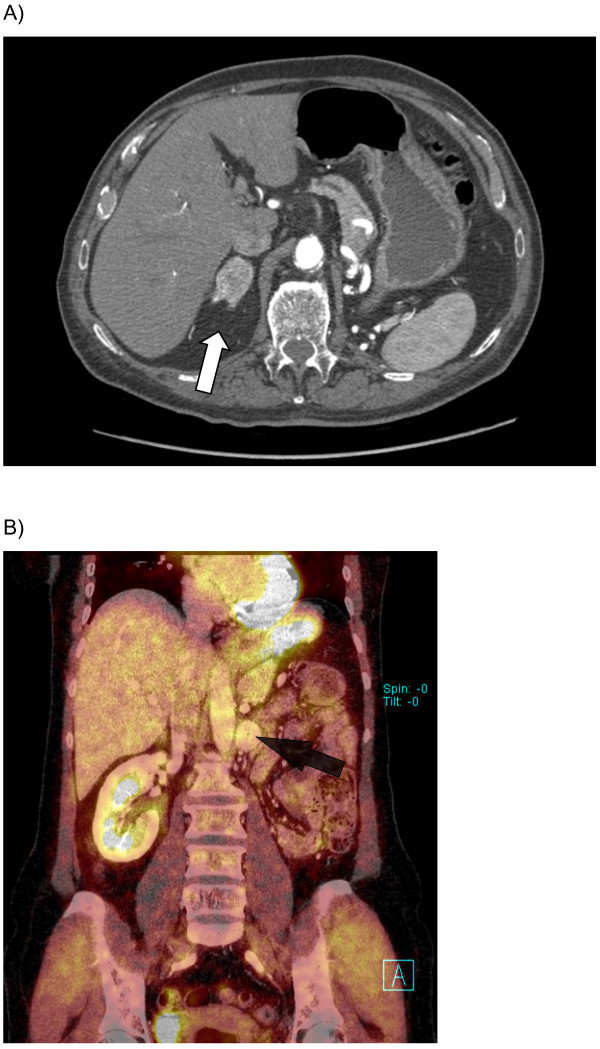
CT (A) and PET-CT (B) scan of patients with ipsilateral metachronous metastasis after radical nephrectomy on the right (A) and left (B) side. Arrows (white and black) mark the corresponding suspicious lesion.
